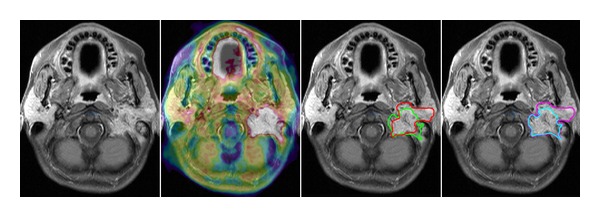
A 47-year-old female patient (patient no. 2) with a recurrent auditory canal cancer located at the base of the skull. FET-PET shows infiltration into the left parotideal gland to a lesser extent than assumed from the MRI. The MRI/CT volume is delineated in red and the PET volume in green. The additional PET information is included in the initial treatment fields (magenta). The boost volume could be reduced as delineated in turquoise.
